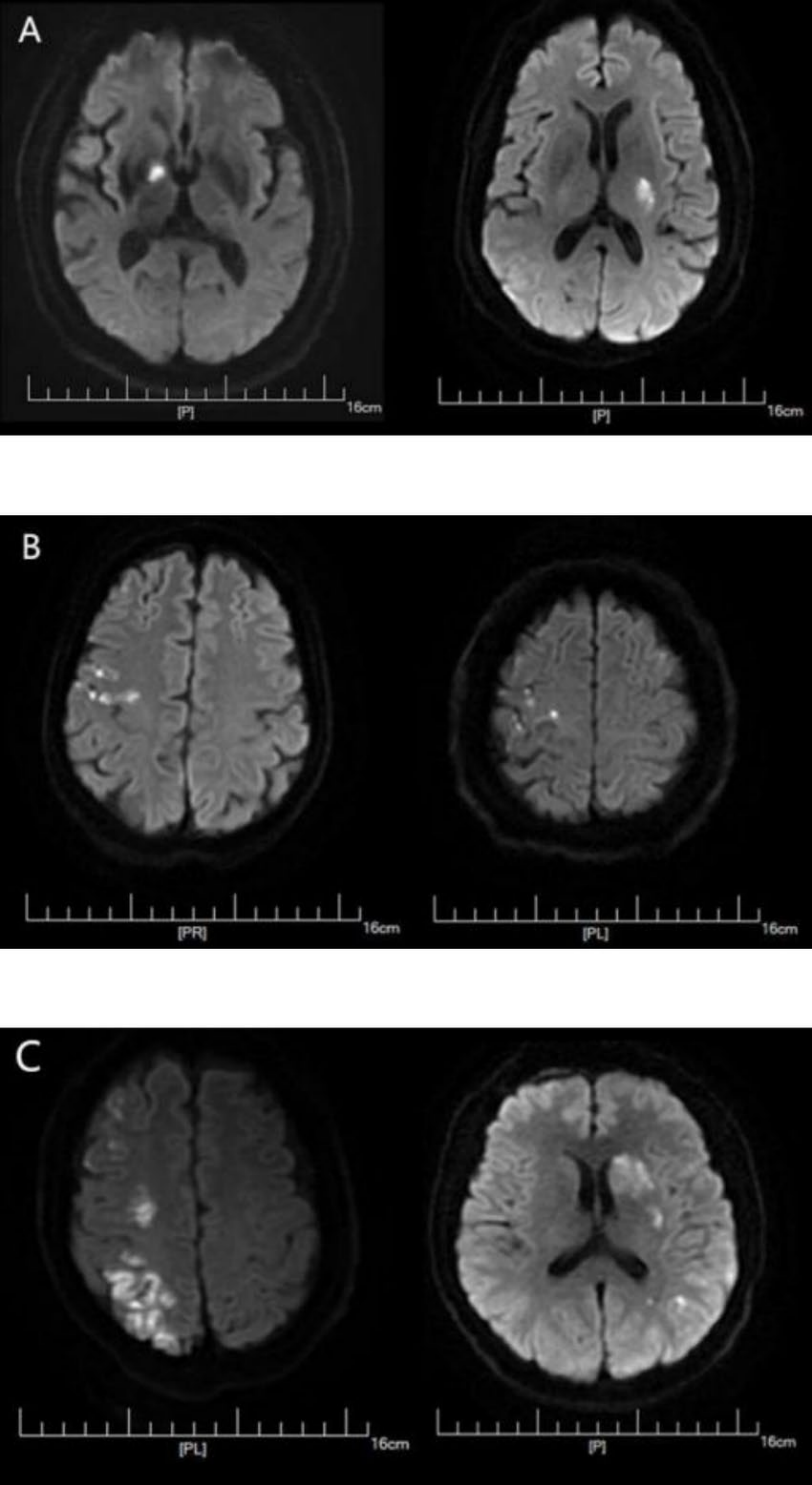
DWI lesion patterns: (A) deep-only pattern, (B) small cortical-only, (C) large cortical/cortical–deep pattern. The hyperintense areas in the DWI sequence images above represent the regions of acute cerebral infarction.
